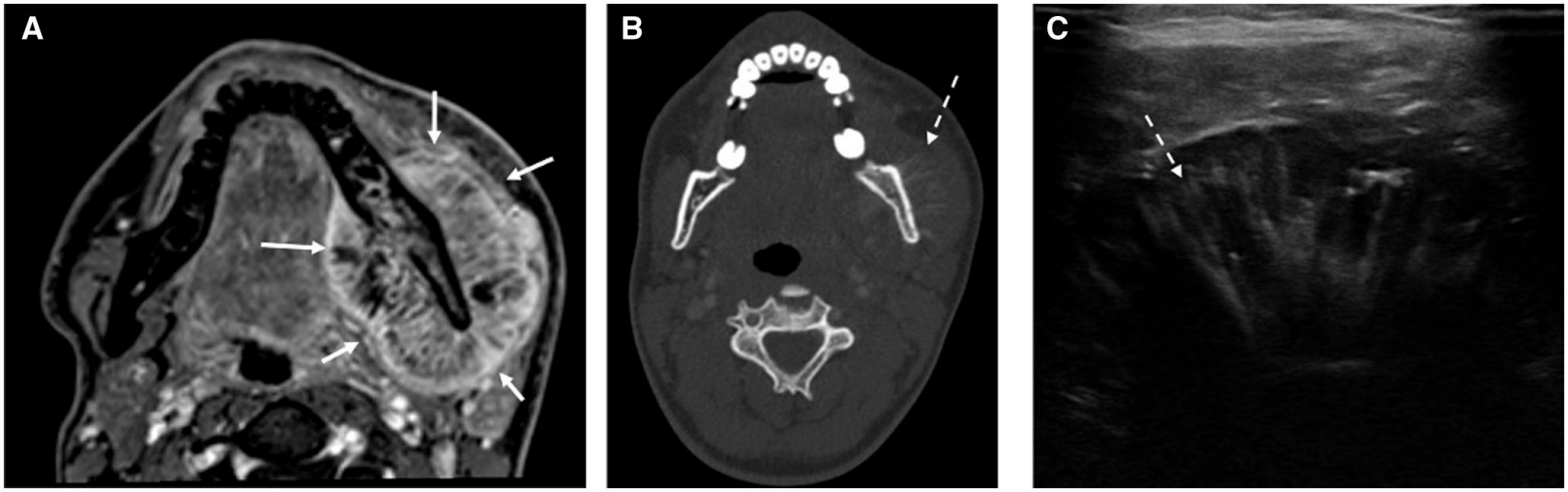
Osteosarcoma of the left mandible. (A) Axial fat-suppressed post-contrast T1-weighted MRI shows an aggressive soft tissue lesion (white arrows) centred on the left mandible that enhances heterogeneously. Intralesional low internal signal reflecting its osteoid matrix, differentiating this from EMPs. (B) Axial CT (bone algorithm) shows aggressive sunburst periosteal reaction along the lingual and buccal cortices of the infiltrated sclerotic left mandible (dotted arrow). (C) US of the left mandible shows heterogeneous soft tissue with linear hyperechoic periosteal reaction. Note the similar appearances of Figures 10B and C and 11B and C, which highlight MRI as a crucial modality in differentiating EMP from osteosarcoma.
